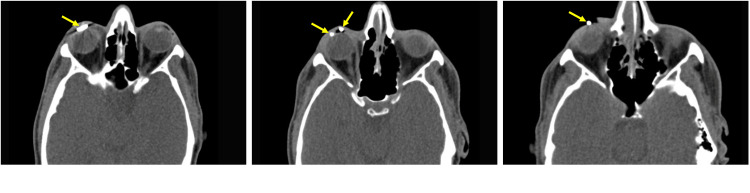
Transverse CT Head Series Showing Fish Hook Lodged in the Anterior Chamber of the Eye Yellow arrows indicate the fish hook in the anterior chamber of the eye, represented as a white opacity.
